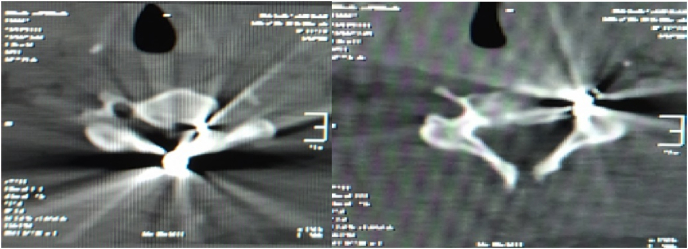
Axial plane of cervical CT scan depicting the position of the pellet fragments.
